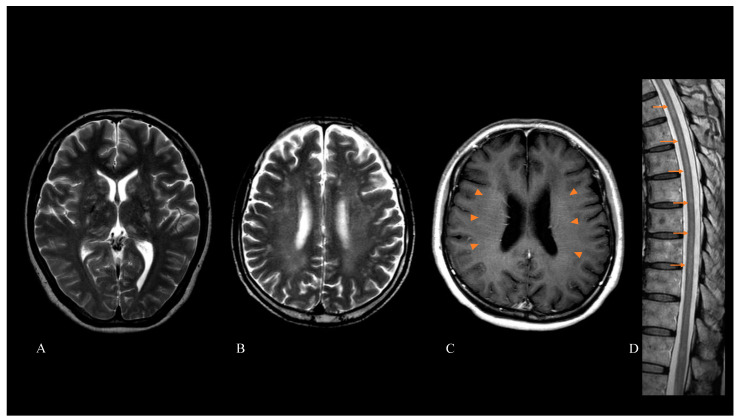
Brain and spinal cord magnetic resonance imaging (MRI) of patients with autoimmune glial fibrillary acidic protein (GFAP) astrocytopathy. Abnormal hyperintensity lesions on T2-weighted imaging were observed in the basal ganglia, thalamus (A), and cerebral white matter (B). Gadolinium-enhanced brain MRI showed a linear perivascular radial enhancement pattern (arrowheads) (C). Spinal cord MRI showed longitudinally extensive T2-hyperintensity lesions (arrow) (D).
